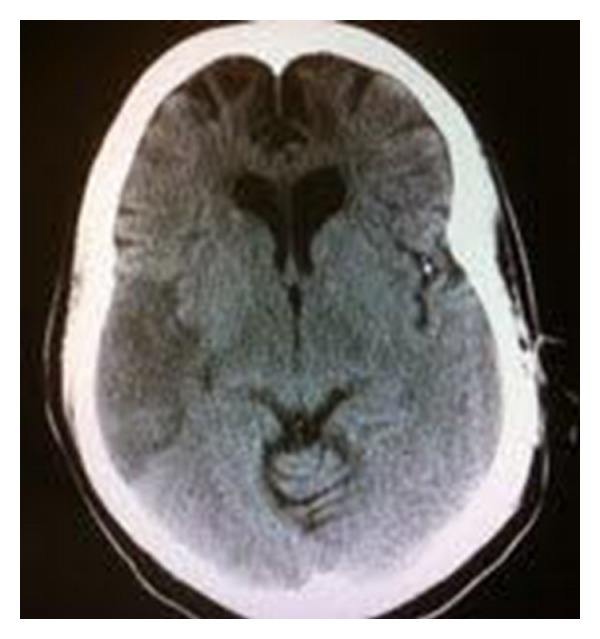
CT scan of the head without contrast showing a larger right MCA infarction compared Figure 1.
